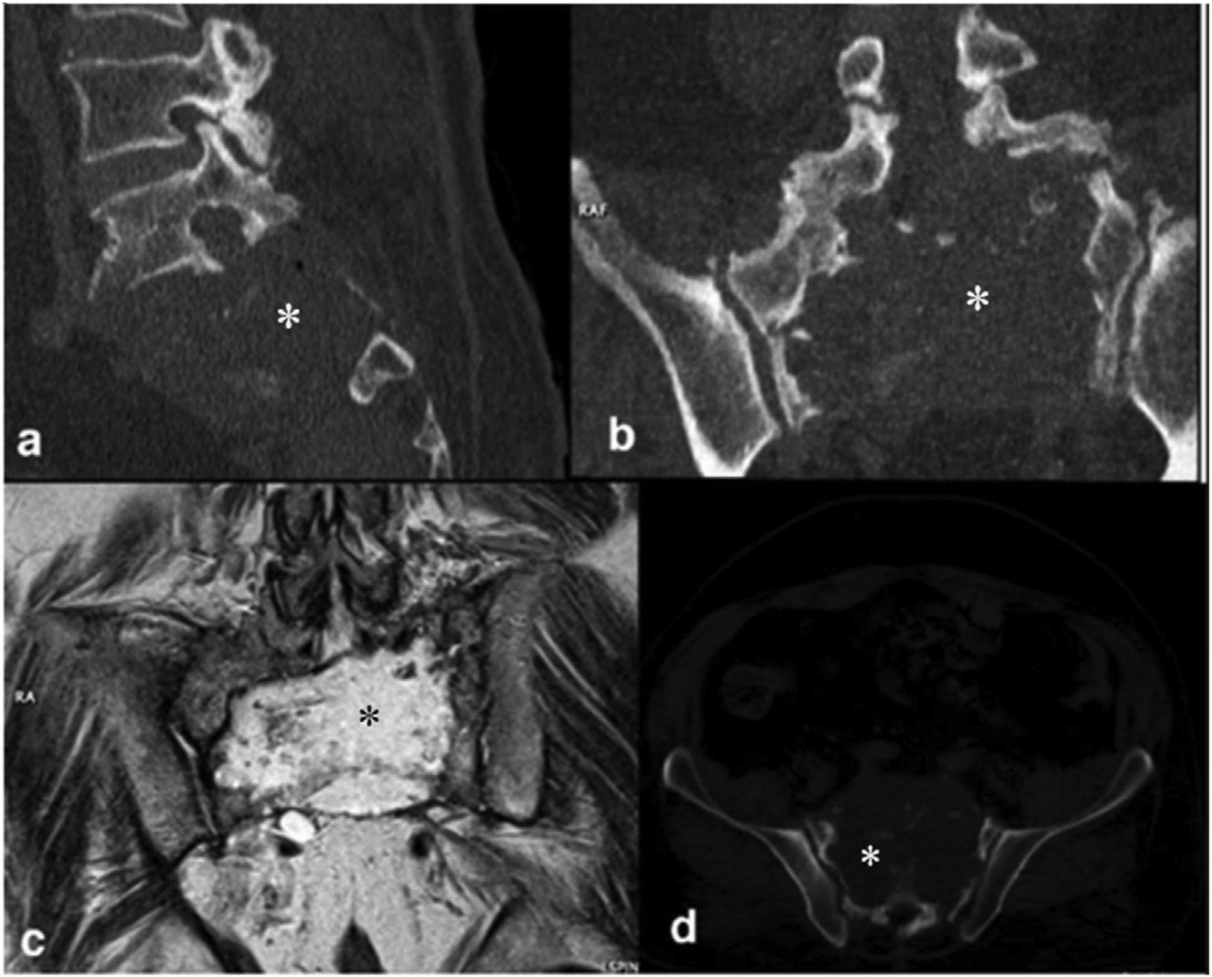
Sagittal multiplanar reconstruction CT scan (a), coronal CT scan (b), coronal T2-weighted MRI (c) and coronal reconstructed CT scan in bone window (d) show a cystic multiloculated hydatid disease in the sacrum (Asterisk). (Photos are from our own patient(s) and they had not been published previously elsewhere.).
